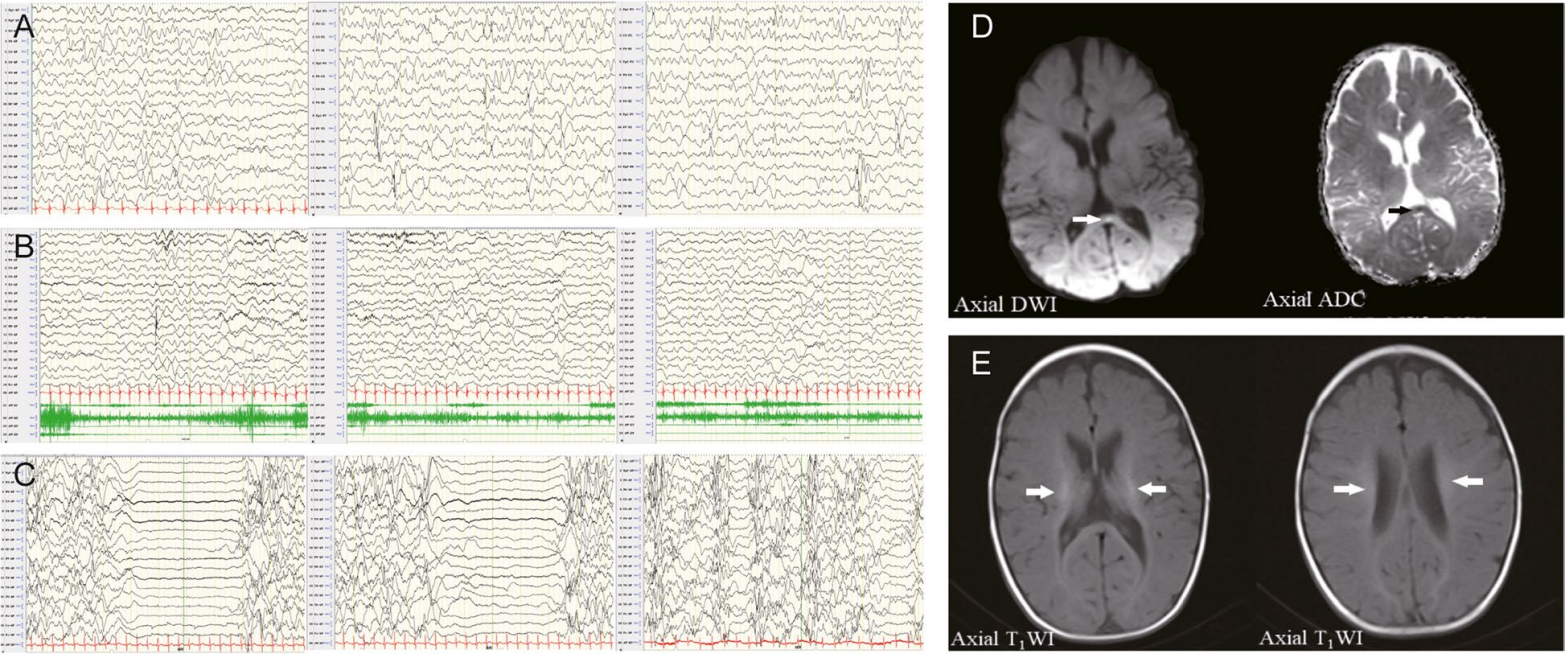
EEG and MRI results in patient with *NARS2* heterozygous variant. A EEG results showed multiple foci-spikes, spike waves, and sharp waves when patient was 3 months and 20 days old. B The patient exhibited upward rolling of the eyes and salivation, while the EEG shows synchronization with low to medium amplitude fast wave rhythm in the left frontotemporal. C The EEG presented highly irregular burst suppression patterns when patient was 4 months and 16 days old. D At the age of 3 months and 12 days, the diffusion-weighted image (DWI) revealed a small and slightly high signal shadow in the corpus callosum. while an apparent diffusion coefficient map (ADC) showed a slightly low signal intensity. E At the age of 4 months and 15 days, the T1-weighted image showed abnormalities in bilateral symmetry signal, and decreased white matter in bilateral cerebral
